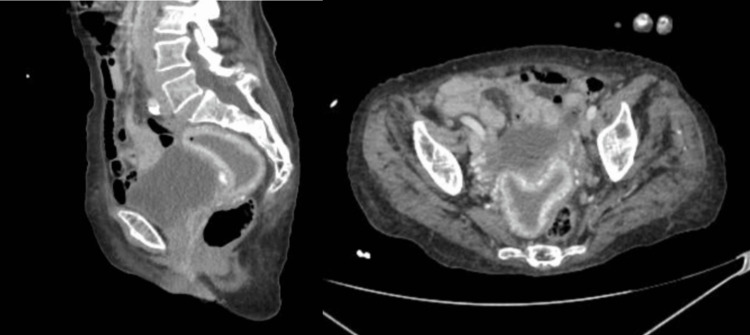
CT scan: endometrial cavity with hypodense content showing the presence of air bubbles and myometrial hyperemia CT: computed tomography
